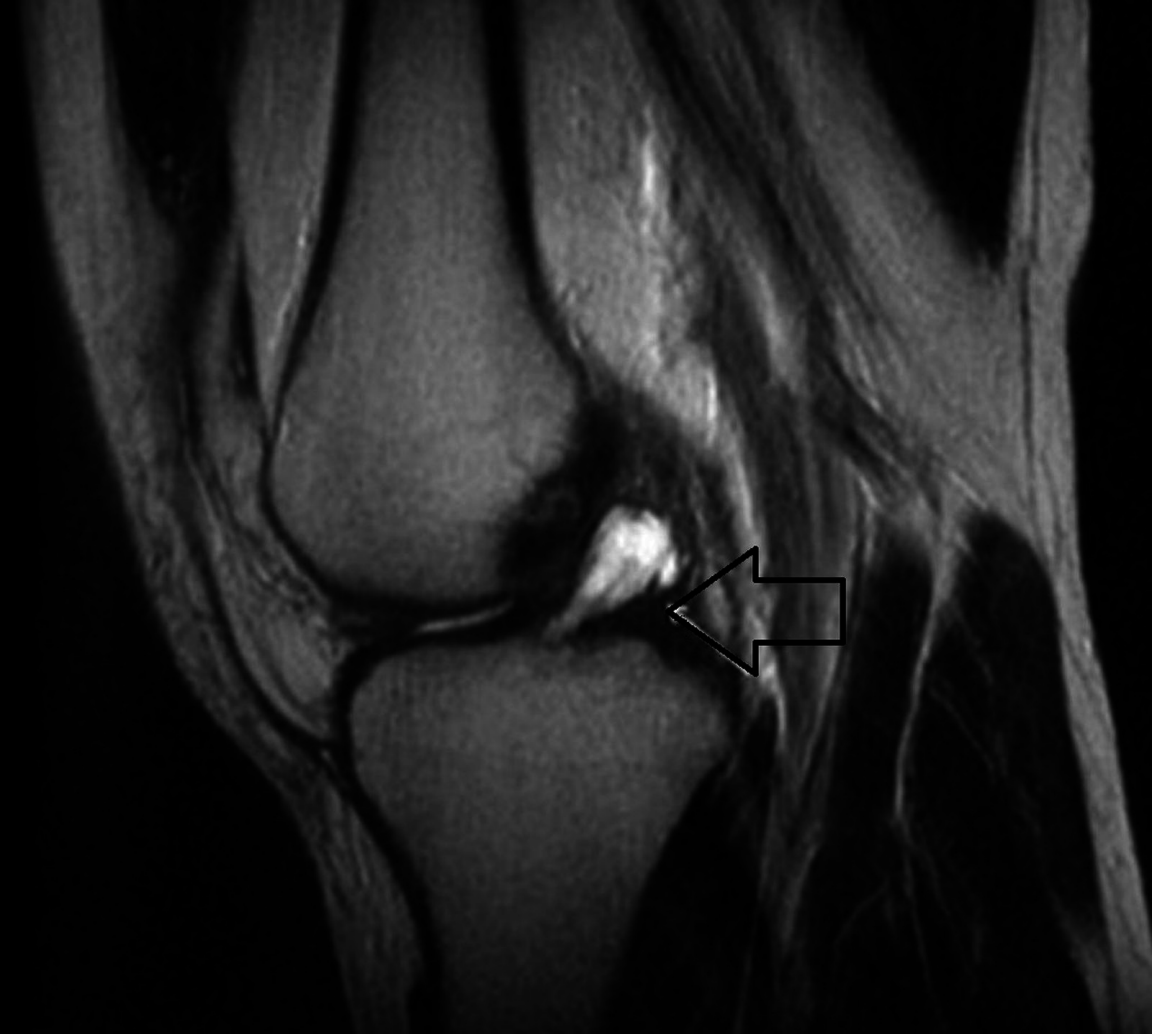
Sagittal T2-weighted magnetic resonance image showing fusiform mass (arrow) that is almost homogeneously hyperintense in the anterior cruciate ligament (ACL).
